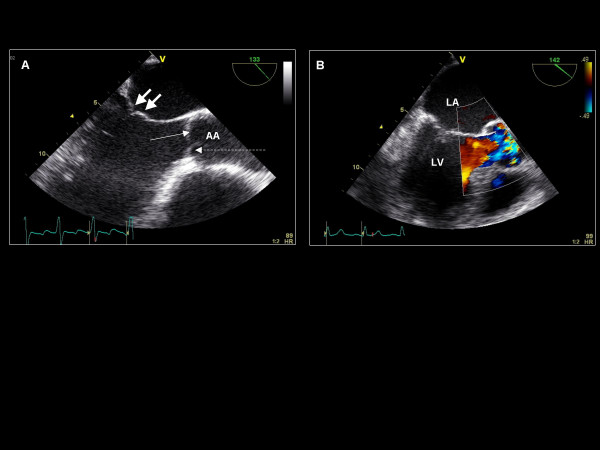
Imaging plane ~135°, (A) The aortic valve and left ventricle long axis view with the left or non-coronary leaflet (solid thin arrow) and right coronary cusp (dashed arrow) of the aortic valve and A2 and P2 scallops of the mitral valve (thick arrows). The ascending aorta (AA) can also be assessed. (B) Color Doppler is applied to the aortic valve and left ventricular outflow tract.
